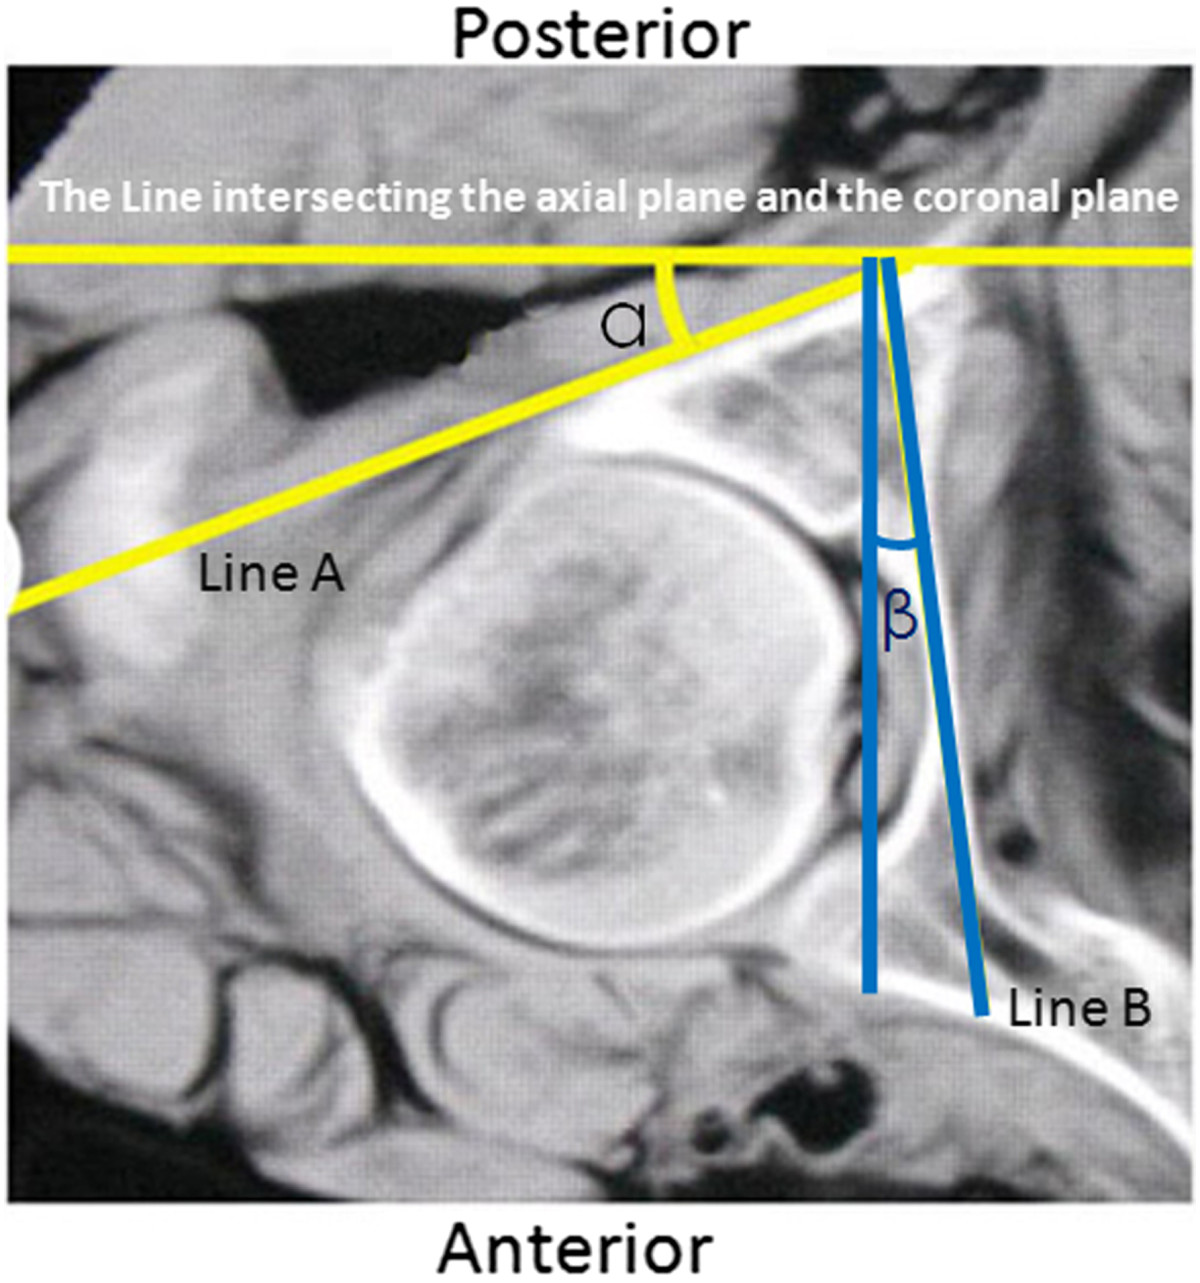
On the axial computed tomography (CT) image at the middle height level of the acetabulum, Line A represents the posterior cortex of the posterior column. The angle between Line A and the line intersecting the axial plane and the coronal plane is labelled angel α. Line B represents the medial wall of the acetabulum. The angle between Line B and the line intersecting the axial plane and the sagittal plane is labelled angle β.
